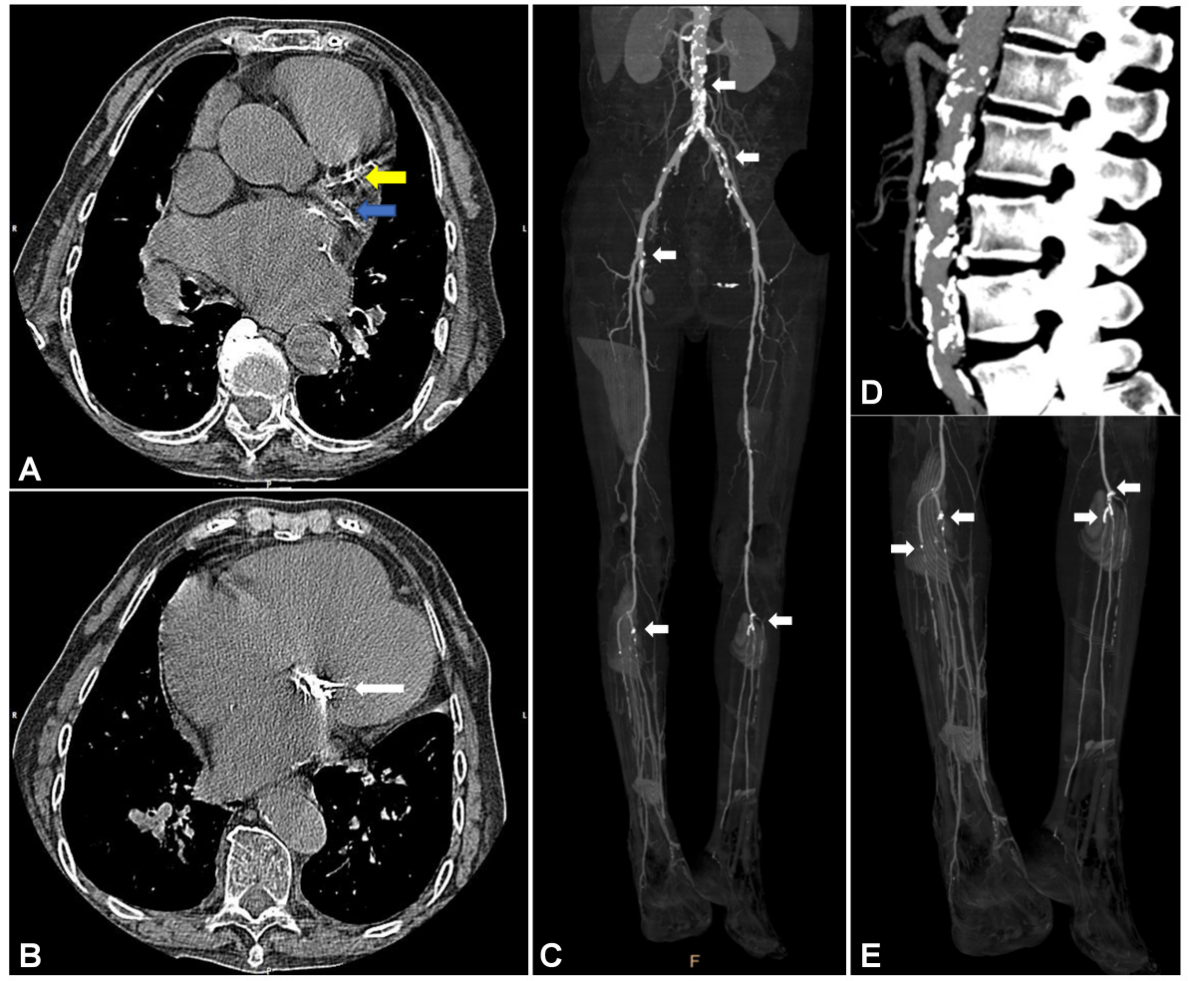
Vascular calcification in cardiovascular vessels and valves. (A) and (B) show vascular calcification in a 74-year-old woman with a history of rheumatic heart disease and hyperuricemia. (A) Representative CT image of vascular calcification in the left anterior descending (LAD, yellow arrow) and left circumflex artery (LCX, blue arrow) of the left coronary artery (LCA). (B) Representative CT image of vascular calcification in the mitral valve. Images (C), (D), and (E) show vascular calcification in a 56-year-old man with a history of arteriosclerosis obliterans (ASO), severe chronic limb ischemia accompanied by intractable and infectious ulcers, and type 2 diabetes mellitus (T2DM). (C) Representative infrarenal CT angiography (CTA) image shows multiple vascular calcifications. (D) Severe vascular calcification in the infrarenal aorta. (E) Severe vascular calcification in the distal popliteal artery and branches with stenosis and occlusion.
